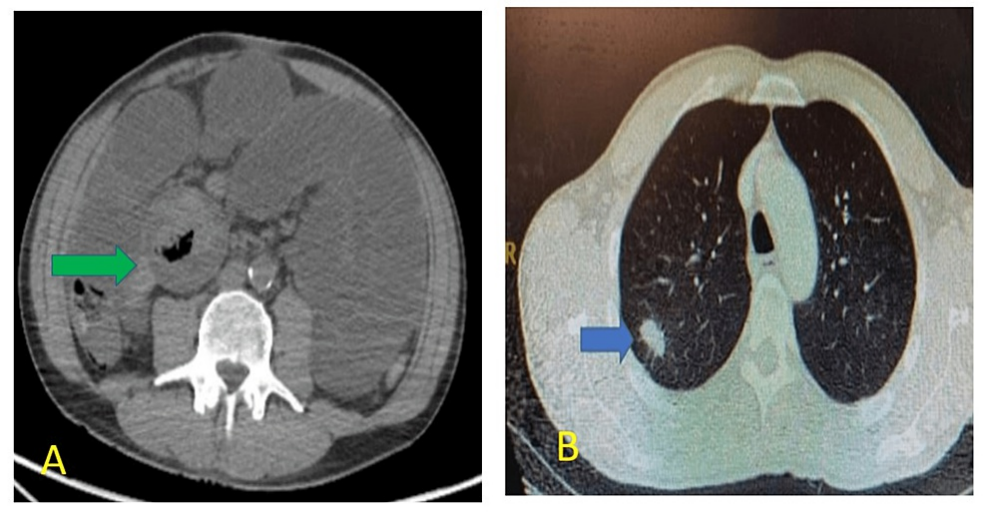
Computer tomography images (A) CT abdomen showing concentric thickening of the jejunal loop (green arrow) along with dilated bowel loops. (B) CT lung showing lung primary tumour in the right upper lobe of the lung (blue arrow).
